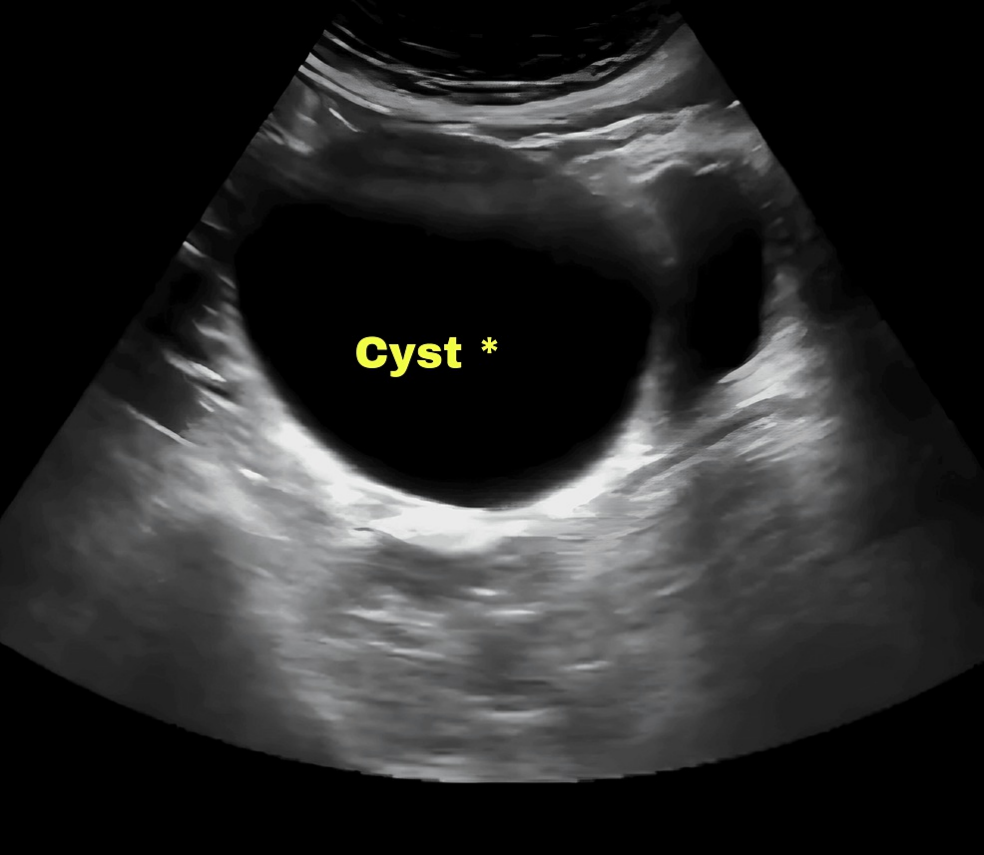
Ultrasound showing the right ovarian cyst of size 10.1 x 6.1 x 5.6 cm
